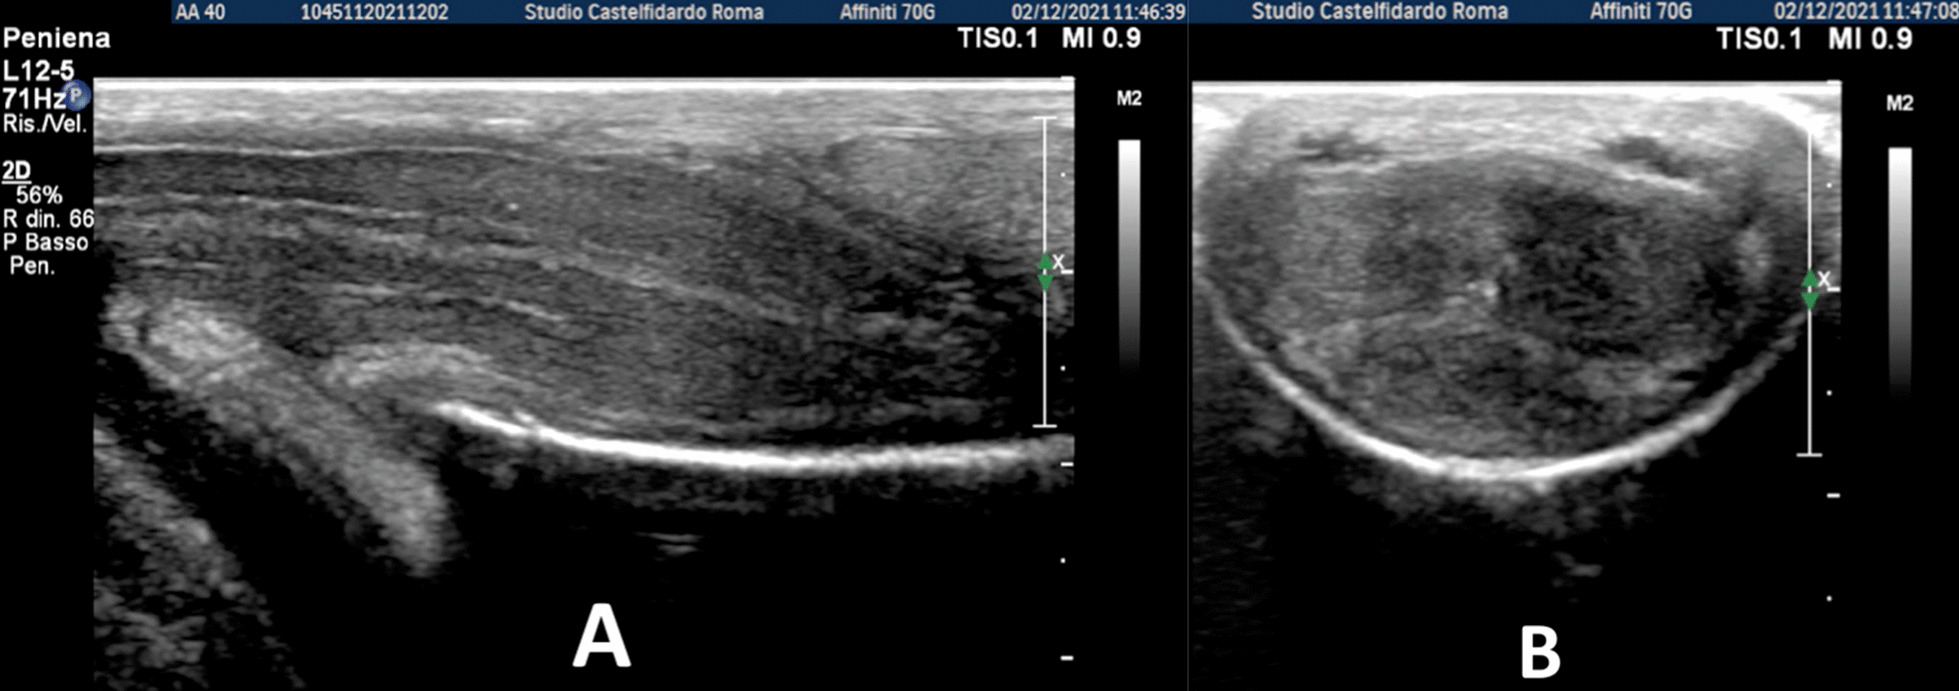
Penile ultrasound after the fourth treatment cycle. A longitudinal scan. B Transverse scan
